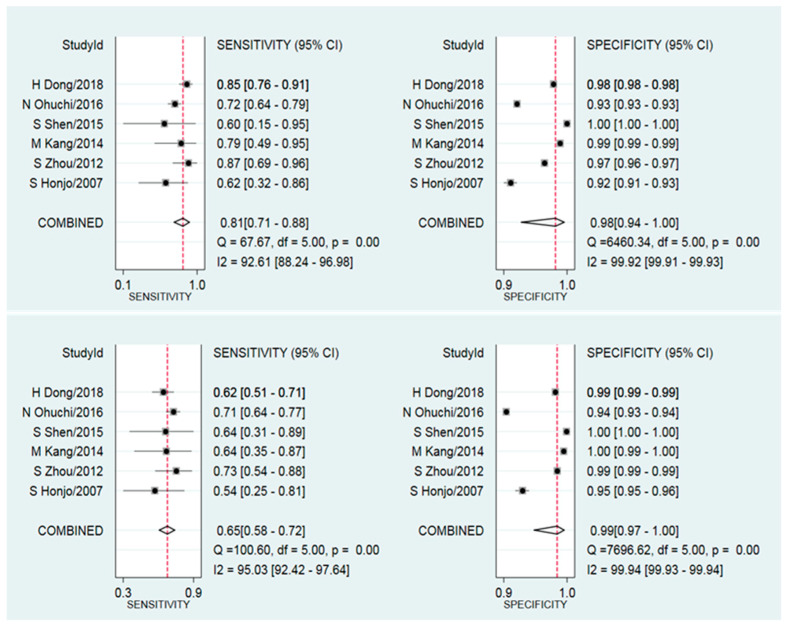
Forest plot of the sensitivity (left) and specificity (right) of mammography (top) and ultrasound (bottom) breast cancer screening in Asian women.
